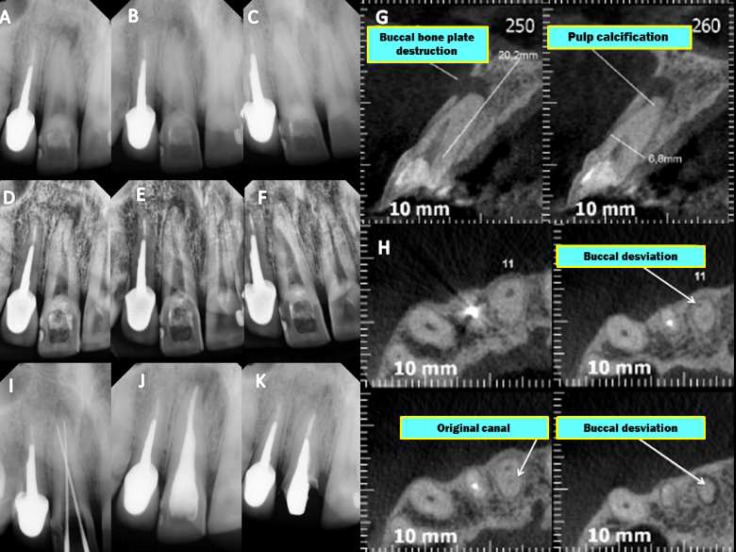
Case 4 *A, B, C)* Initial DR at different angulations; *D, E, F)* Evaluation of DR using the periodontics filter digital system XDR®; *G,H)* CTCB: sagittal and axial slices showing canal deviation identified buccally (ARROWS); *I)* DR with the identification of the original canal; *J)* Final radiograph after obturation; *K)* Follow-up radiograph taken 2 years after treatment
